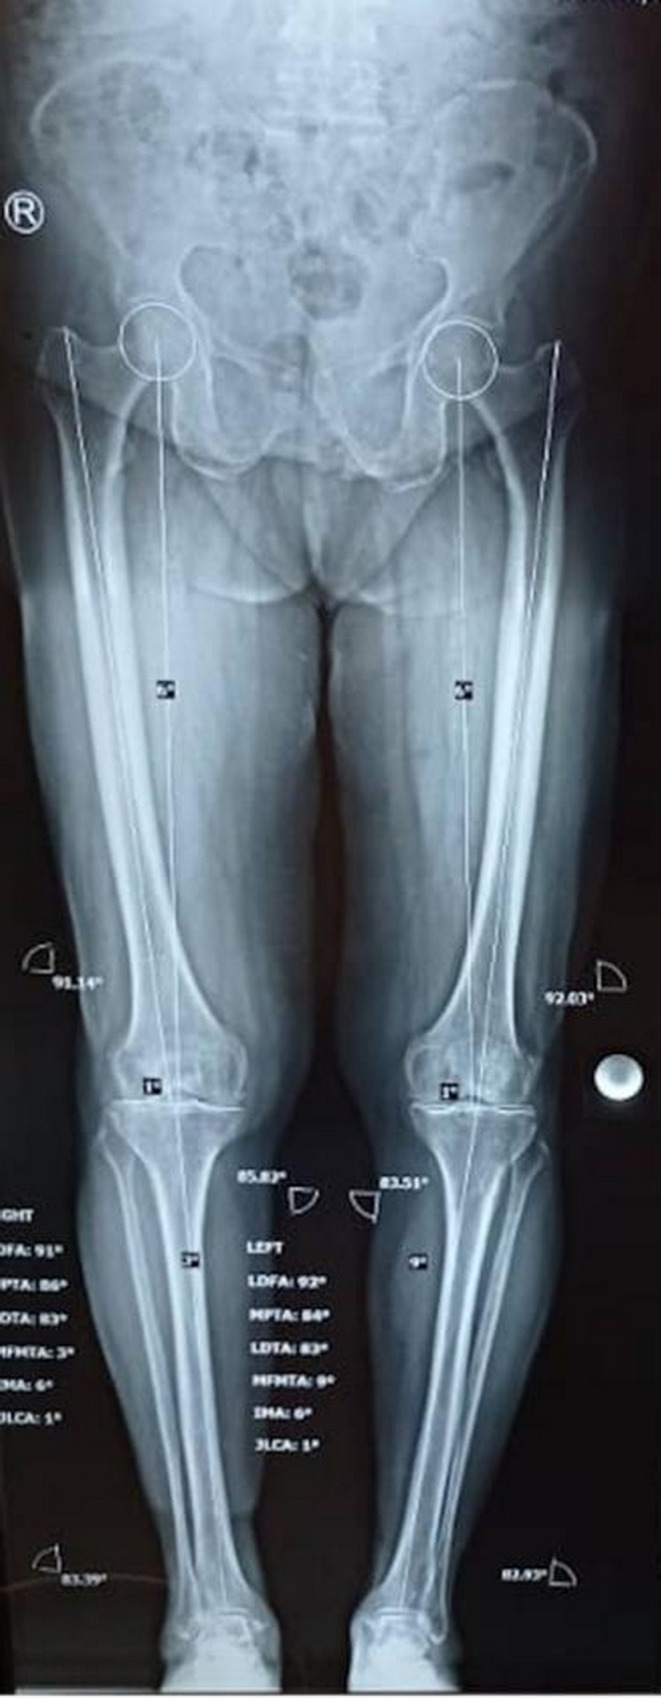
Hip‐knee‐ankle radiographic with joint space narrowing, subchondral sclerosis, and osteophyte formation.
